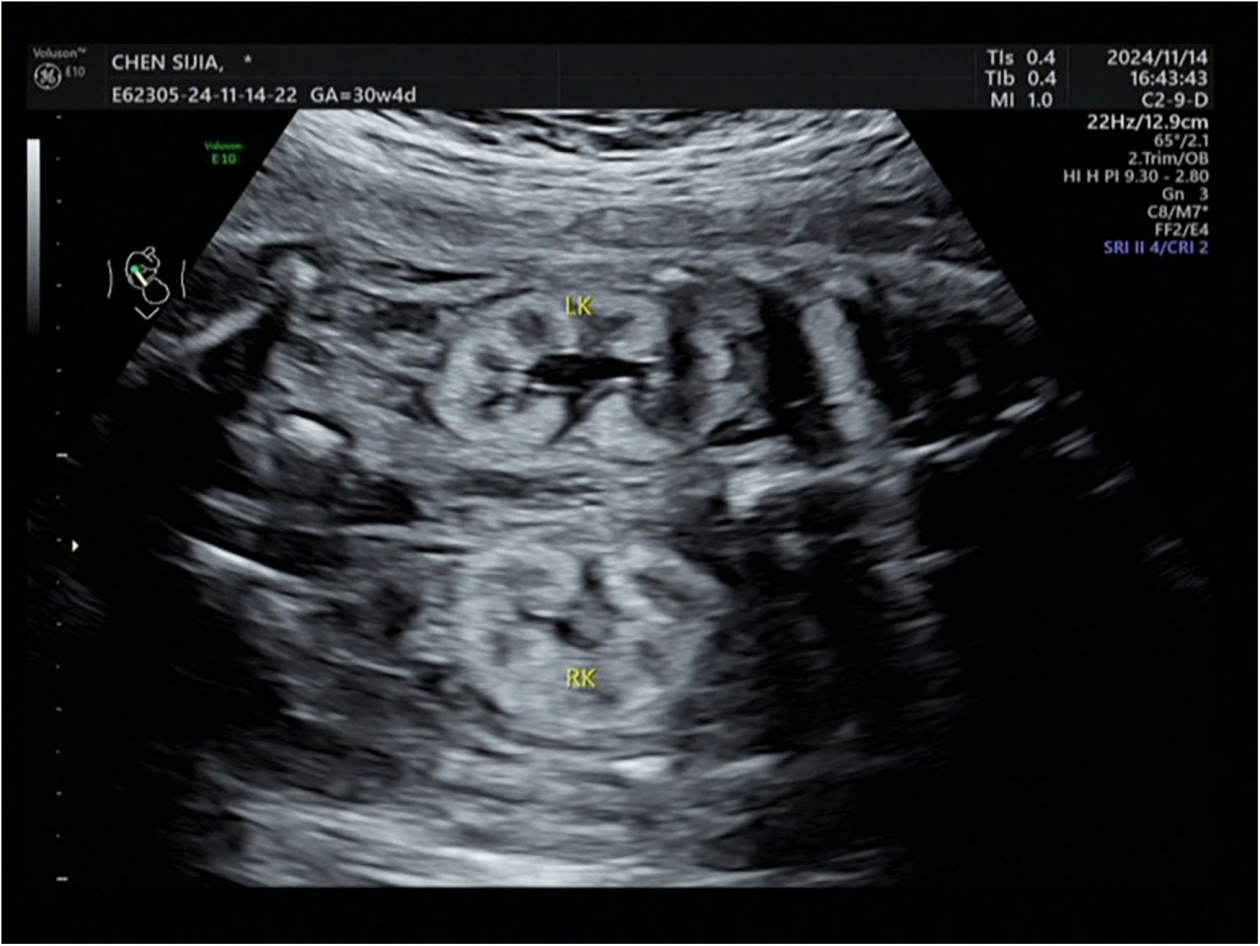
Intrauterine ultrasound phenotype of fetal hyperechoic kidney.
